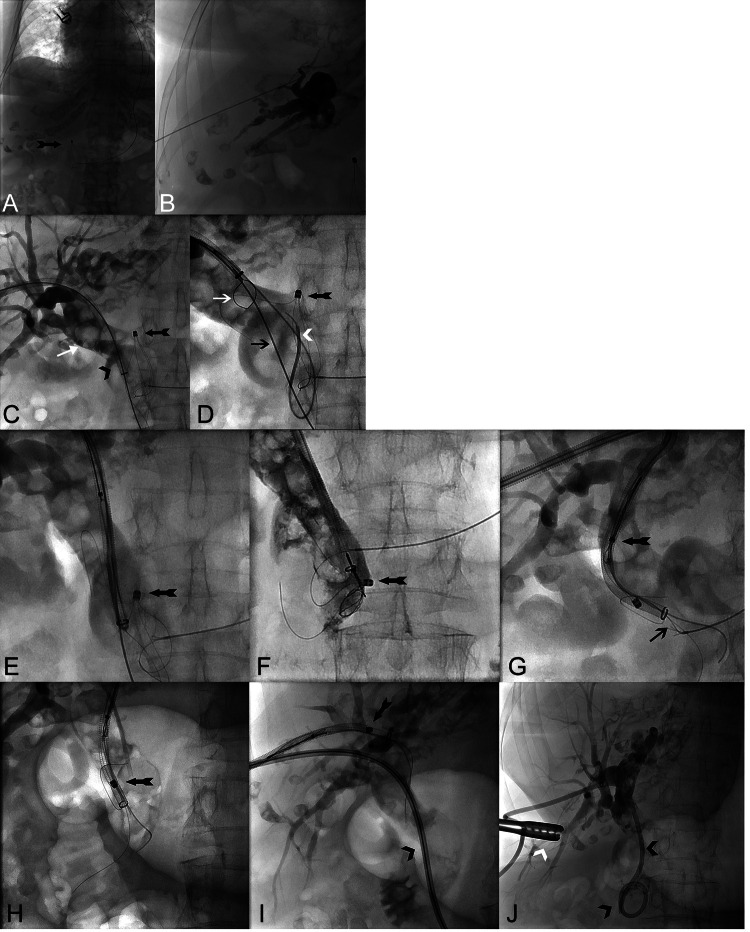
(A-D) (A) Severed DB (black arrow) impacted in the CBD with the pulling-wire. (B) Ultrasound-guided percutaneous transhepatic biliary approach (Neff Percutaneous Access Set™, Cook, USA) into the right-sided biliary system in the angio-suite. (C and D) Following the insertion of a 10Fr sheath (Arrow®, Teleflex, USA, black arrow head), recanalization into the duodenum was achieved using a 0.035′ wire (Radifocus™, Guide Wire, Terumo, Japan) and a 4F catheter (Radifocus™, Glidecath™, Terumo, Japan, white arrow head). An 0.035′ heavy-duty wire was subsequently placed as a support wire (Amplatzer wire, BSCI, USA, black arrow) before attempts were made to capture the DB. The imaging reveals intrahepatic bile ducts containing several small and larger stones (white arrow), with the DB (black feather arrow) situated in the CBD. Unfortunately, the metallic tip of the DB had already perforated the CBD. The combination of the DB tip perforation and the presence of large concrements inside the DB elucidates why the endoscopic removal proved unsuccessful. (E-G) (e and f) To mobilize the DB and its metallic tip embedded in the CBD wall, various goosenecks (Amplatz™, Medtronic, USA) in three different sizes and versions with one to three loops were attempted. However, only through the combination of a 4F Catheter (INFINITI®, Cordis™, USA) and an EN-snare endovascular system (ONE Snare®, Merit Medical, USA), the successful mobilization of the DB at the distal end and its retraction into the 10F sheath was achieved (black feather arrow). (g) The final two remaining holding wires connected to the DB were endoscopically cut (black arrow). (H-J) (H and I) The DB, snared by the gooseneck, was successfully drawn entirely into the 10F sheath (black feather arrow). The sheath was then completely removed through the percutaneous transhepatic approach. (J) With the help of a large tong (white arrow head) and in it folded 3 sterile pieces of gauze, we were able to close the access channel after 15 minutes of manual compression. Because of the large 10F approach and because of the perforation of the CBD by the tip of the basket, a new 8.5F PTBD (black arrowhead) over the right-sided bile duct was placed.
